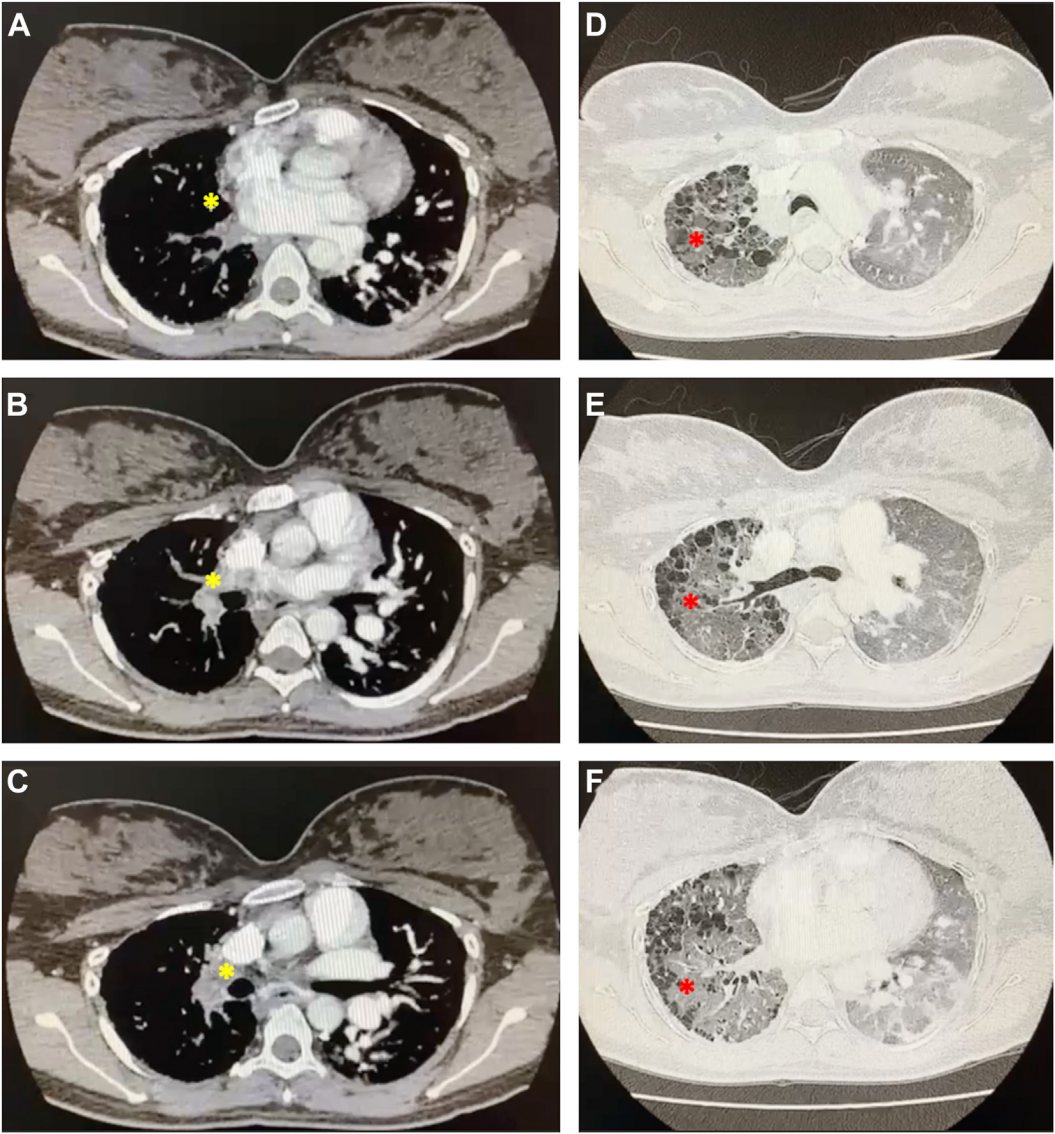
Chest CT with contrast, serial axial images from caudal to cranial (A-C), demonstrates filling defects where an RPA should arise from the main pulmonary artery trunk (*yellow asterisks*). (D-F) Using the lung window, there is evidence of a hypoplastic right lung with multiple clustered lung cysts of peripheral and peribronchovascular distribution (*red asterisks*), with left lung compensatory hyperinflation, pulmonary plethora, and focal consolidation of the posterior segment of the left lower lobe suggestive of acute infective changes.
